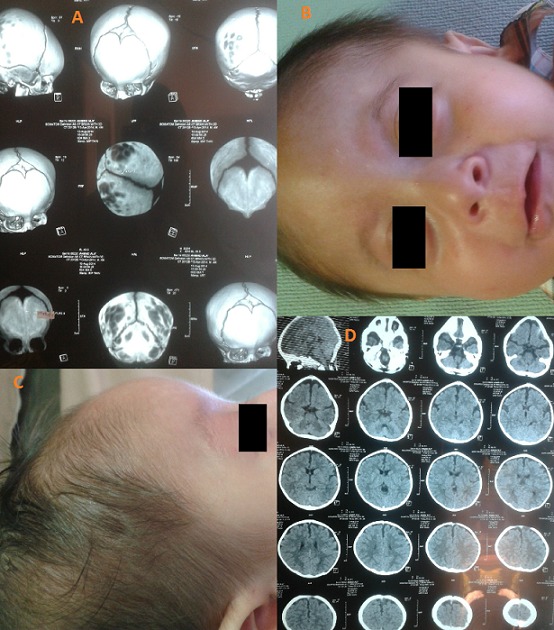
(A) the metopic suture fusion at CT-Scan; (B,C) craniosynostosisat the clinical examination; (D) normal brain parenchyma at CT-Scan
